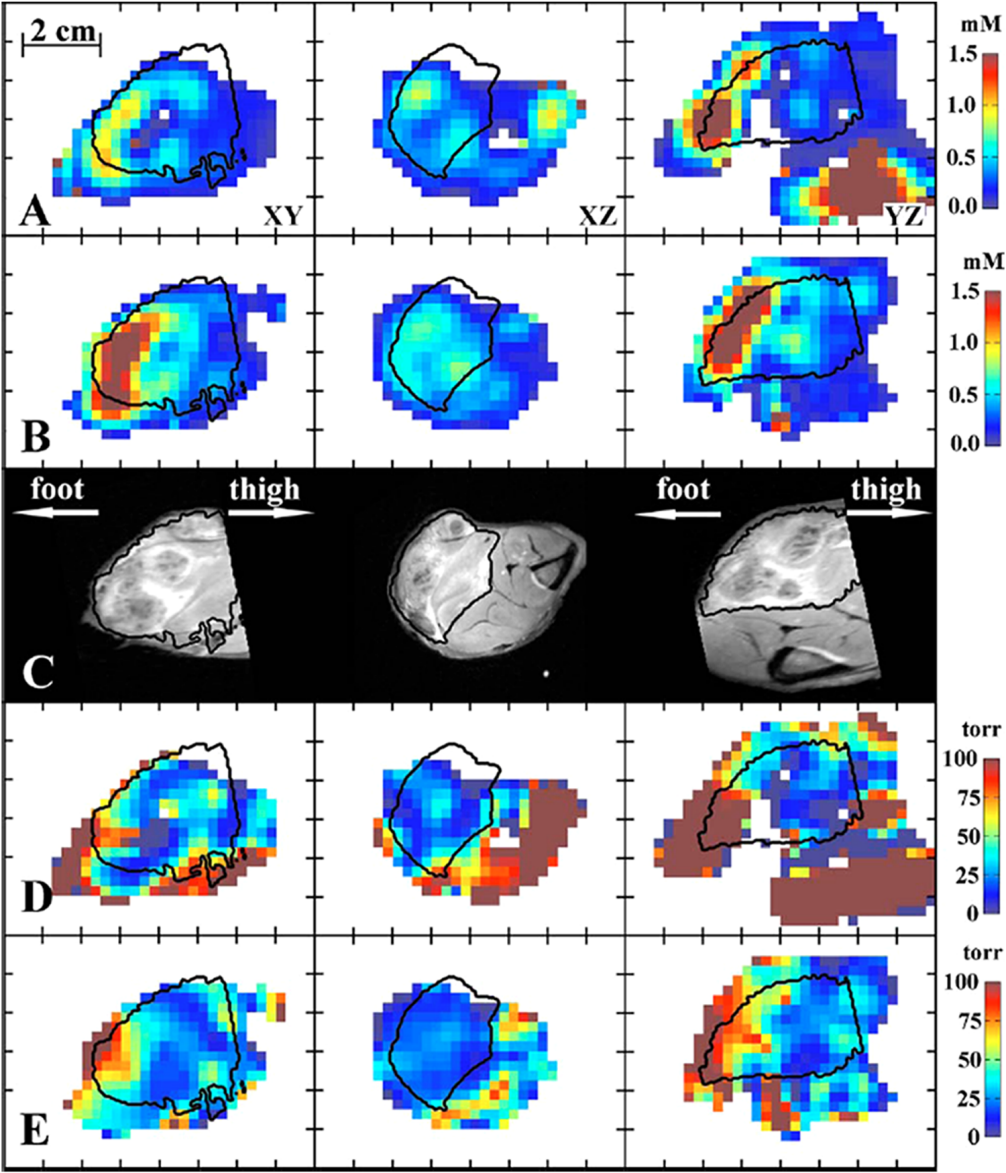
Selected slices of images: a CW concentration, b ESE concentration, c*T*_2_ weighted MRI, d CW pO_2_, and e ESE pO_2_. The tumor region is determined from the MRI image and is outlined by contours (adopted from [34])
